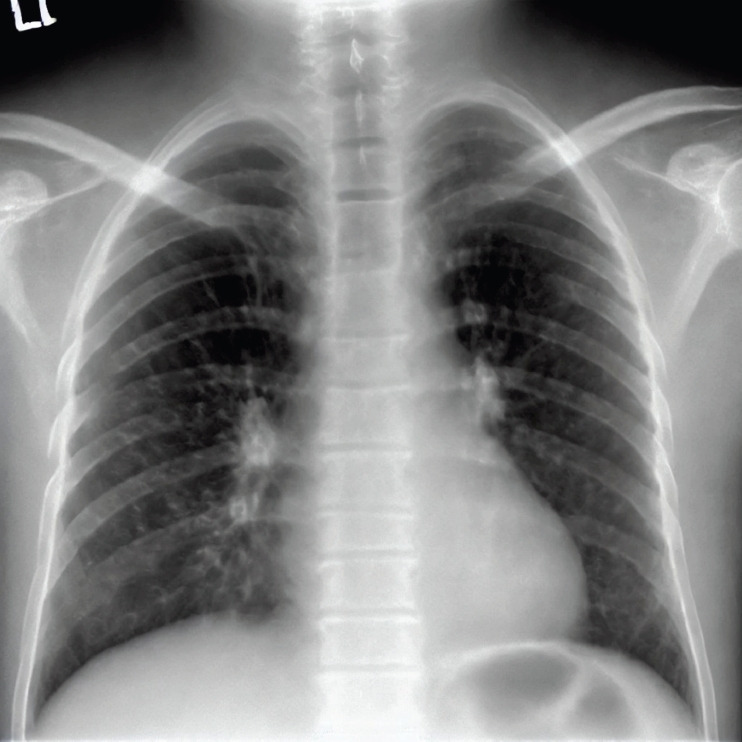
Chest X-ray generated by GPT-4o for objective structured clinical examination station number 2. Generated using the prompt: “generate a chest X-ray showing a right lower lobe pneumonia.” GPT-4o produced an image resembling a chest X-ray but failed to depict alveolar consolidation in the right lower lobe. Notably, several anatomical abnormalities can be observed, including an additional right rib, vertebral compression, and apparent absence of spinous processes.
